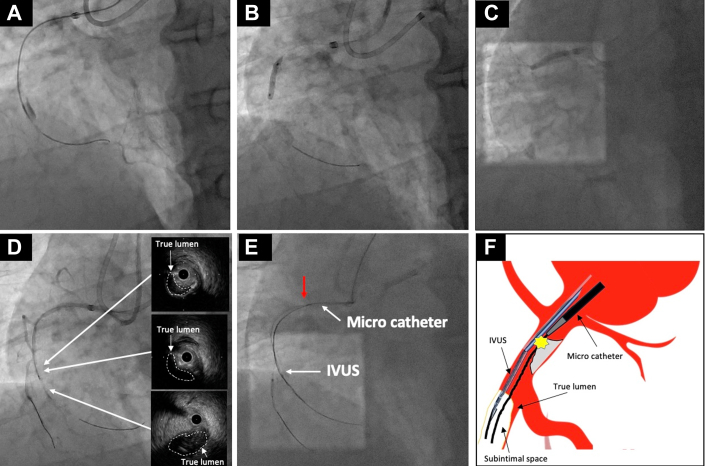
Percutaneous Coronary Intervention (A) In the antegrade approach, neither a Gaia Next2 wire nor a Gladius wire were able to pass through the CTO lesion using angiography. (B and C) POBA was performed for the proximal lesion using a Kizashi 3.0 × 15 mm balloon. (D) We confirmed that the Gladius wire was in the subintimal space distal to the CTO entrance. (E and F) Neither the Corsair-Pro nor the Caravel microcatheter were able to pass through the calcified lesion cracked by POBA near the RCA ostium (red arrow). Fluoroscopy was performed with the adjunctive use of the “SPOT ROI” function^8^ available on the Alphenix Evolve Edition x-ray system (Canon Medical Systems). CTO = chronic total occlusion; POBA = plain-old balloon angioplasty; RCA = right coronary artery; ROI = region of interest.
